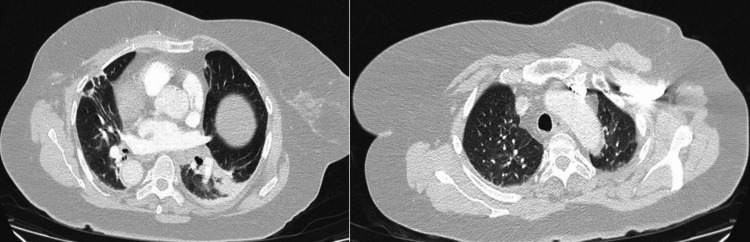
CT pulmonary angiogram with lung windows. Multi-slice images from a CT pulmonary angiogram with lung windows. In the lung windows, foci of consolidation were seen in the left upper lobe and bilateral lower lobes, most severely in the posterior segment of the right lower lobe. CT: computed tomography.
